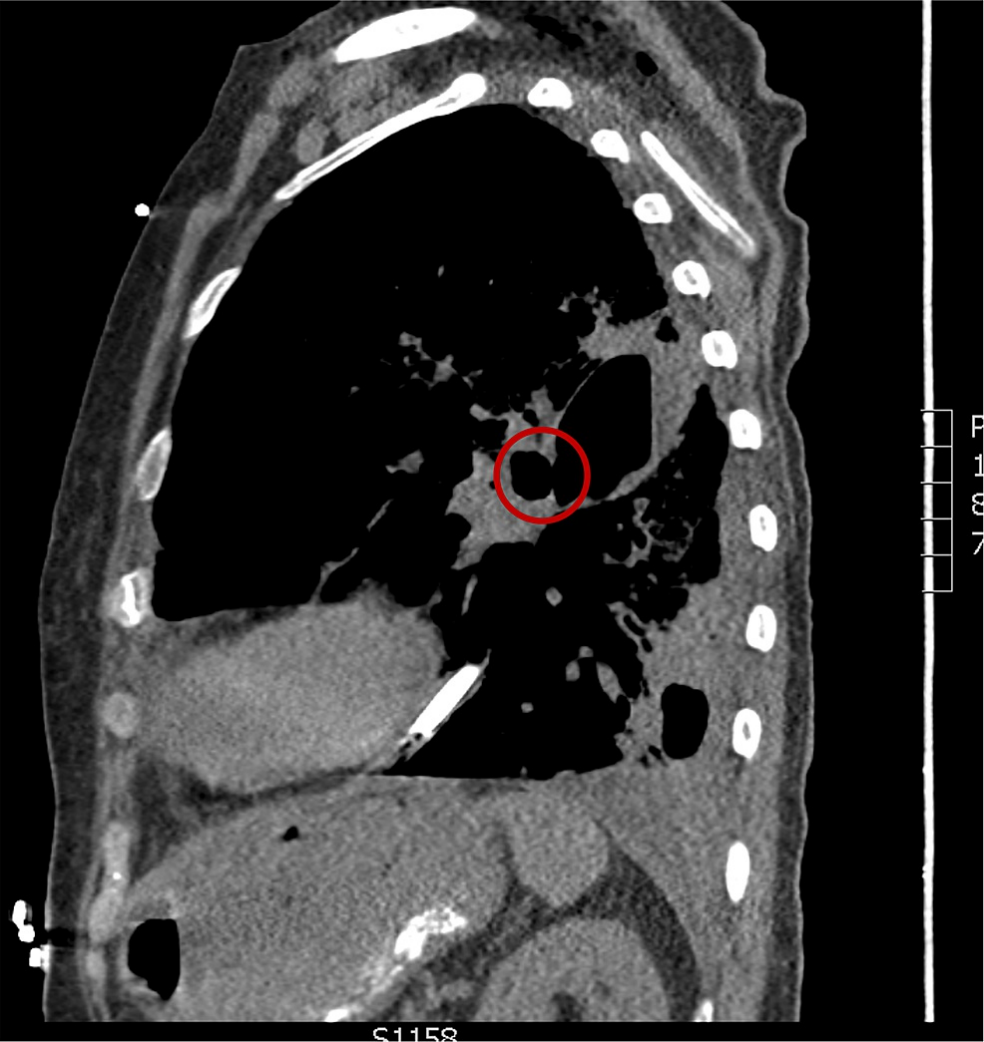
CT chest (sagittal view) showing bronchopulmonary fistula, a cavitary complication of the patient's necrotizing pneumonia
